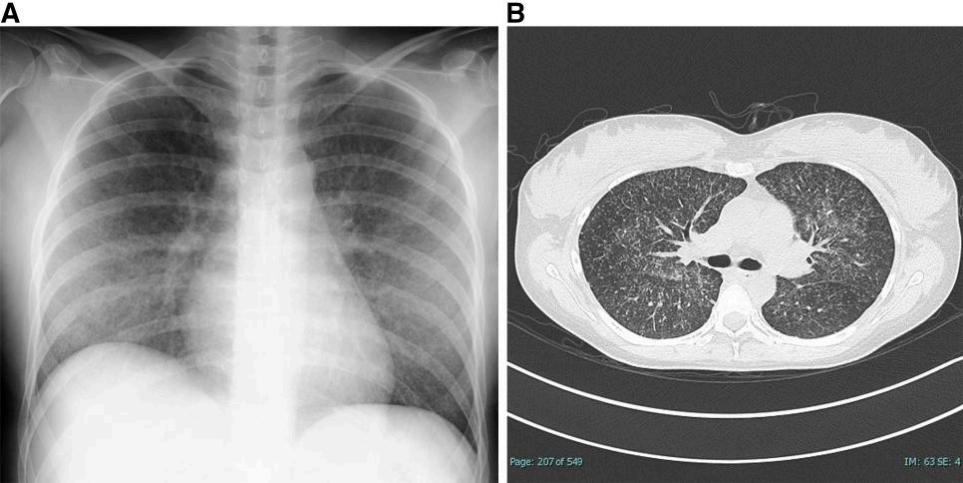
(A) CXR on day 2 showed patchy infiltrates and tiny diffuse nodules throughout the lungs consistent with miliary tuberculosis. (B) Chest CT scan on day 2. Diffuse small granular nodules are seen in the lung fields consistent with miliary tuberculosis.
